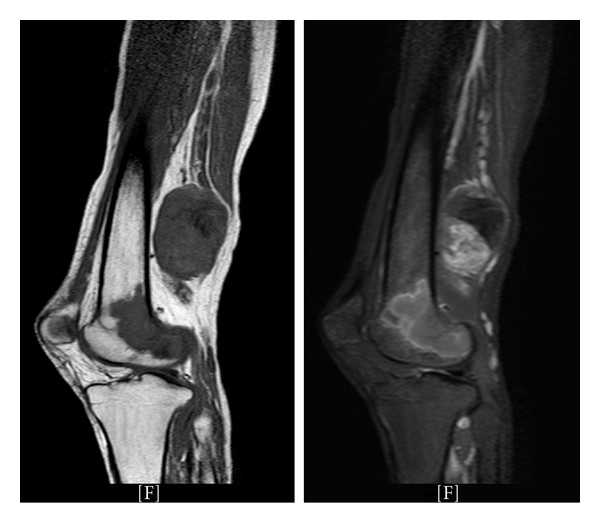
Urgent MRI of the right lower limb; T1 weighted (left), T2 weighted (right). A large (approx. 15 cm), oval, smoothly defined heterogenous mass with a large necrotic centre is clearly visible behind the lower femur (white arrows). There is also an area of altered signal within the femur itself involving the condyle, suggestive of bony metastasis (white arrowheads). The likely diagnosis is malignant peripheral nerve sheath tumor (MPNST) with a differential diagnosis of lymphoma or other soft tissue sarcoma. The character and distribution of the pain could signify a sciatic nerve origin.
